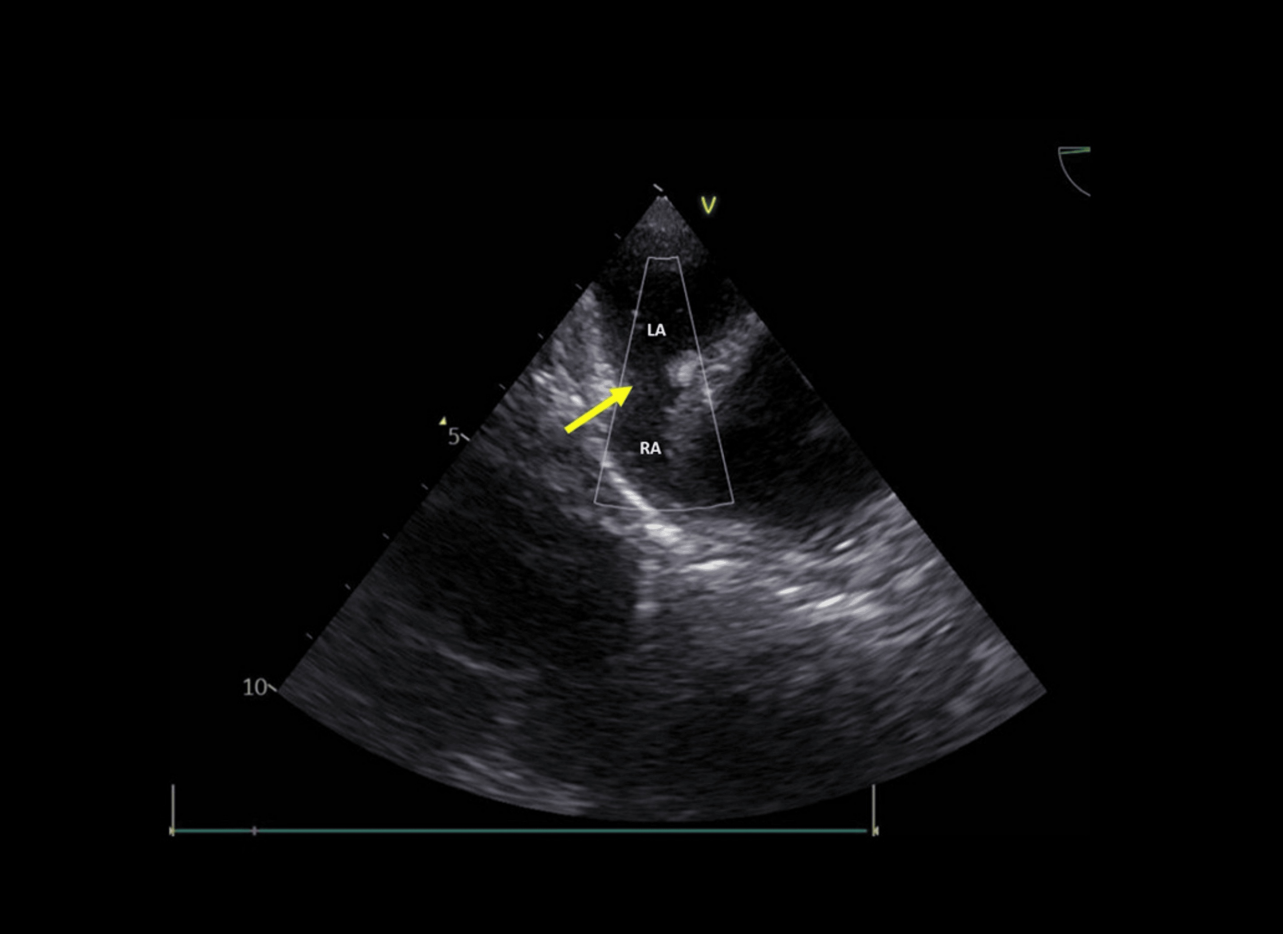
Two-dimensional echocardiogram. RA: right atrium. LA: left atrium. The yellow arrow indicates the patent foramen ovale.
